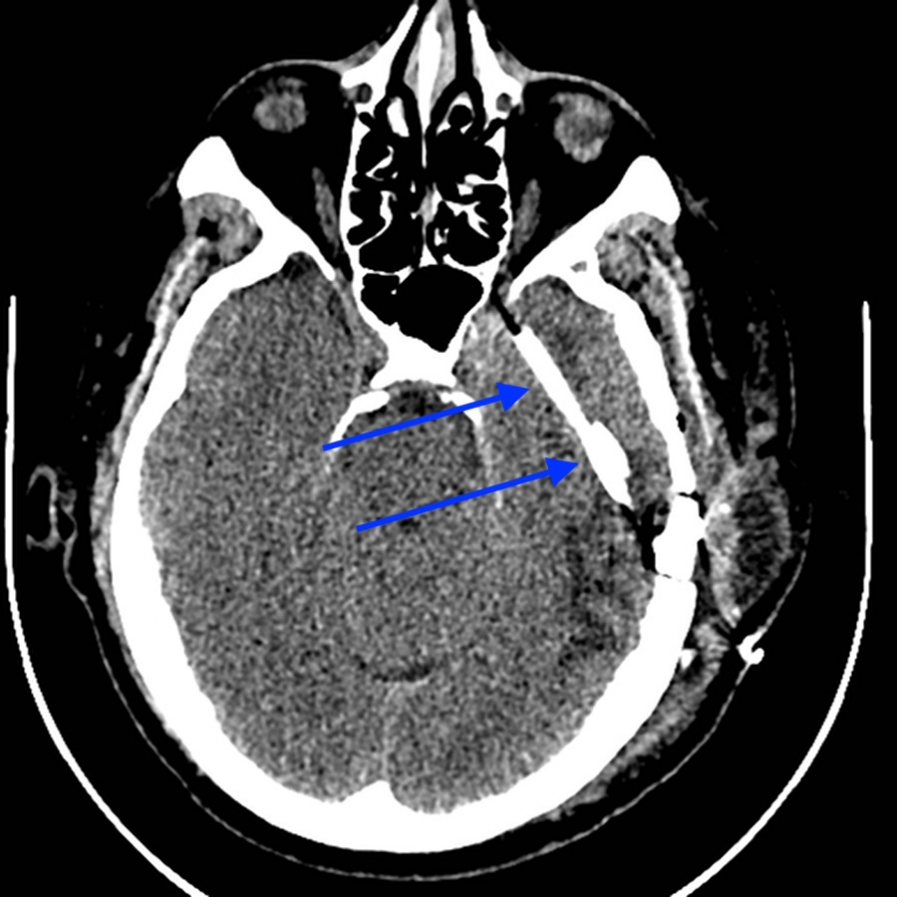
Postoperative unenhanced axial CT of the head: shunt (blue arrows) in situ. CT - computed tomography
